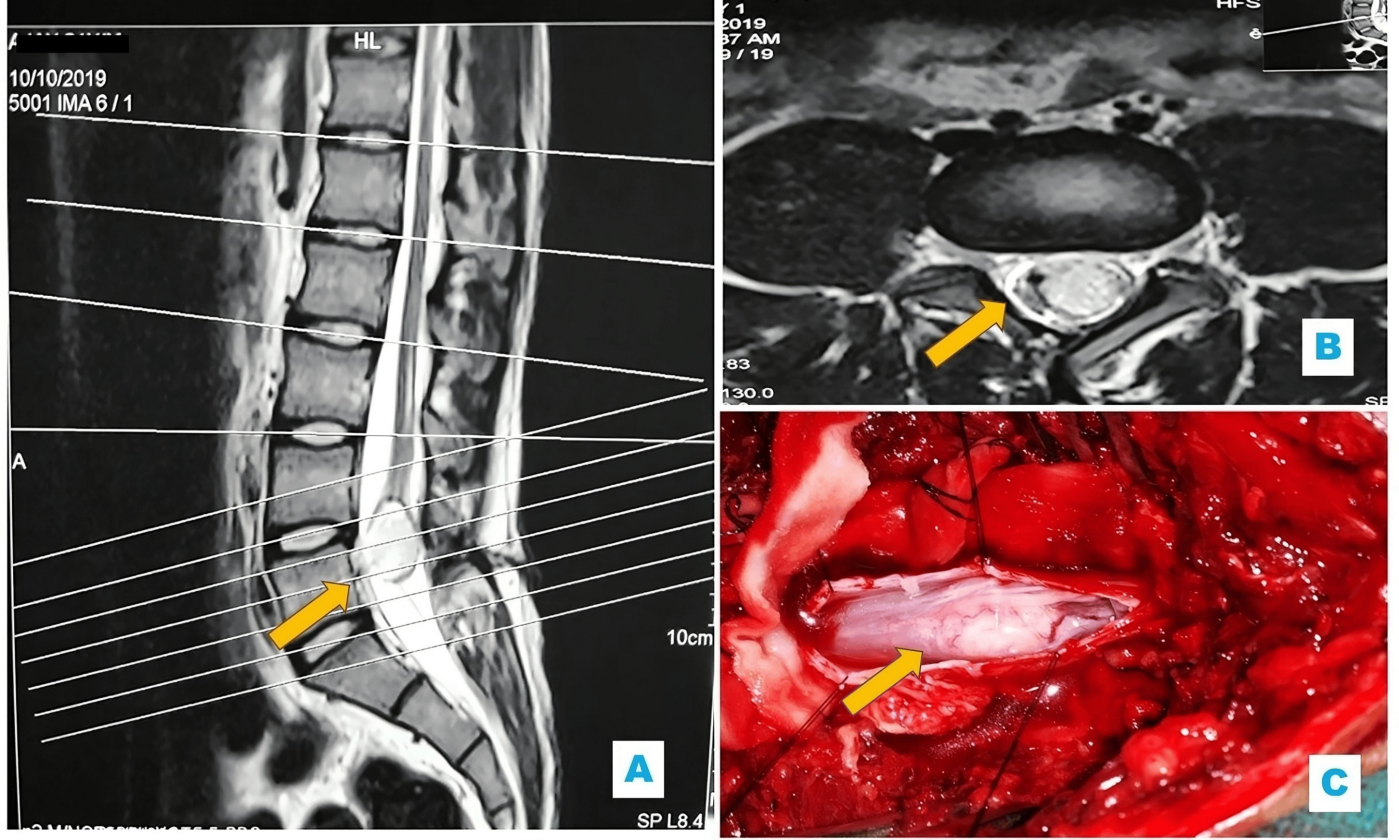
(A) Sagittal T2-weighted MRI demonstrating a well-defined dermoid cyst (indicated by arrow) at the level of the lower lumbar spine, associated with a low-lying conus medullaris. (B) Axial T2-weighted MRI at the same level, showing the dermoid cyst adjacent to the conus medullaris (arrow). (C) Intraoperative photograph revealing the exposed conus dermoid (arrow) following surgical exposure
